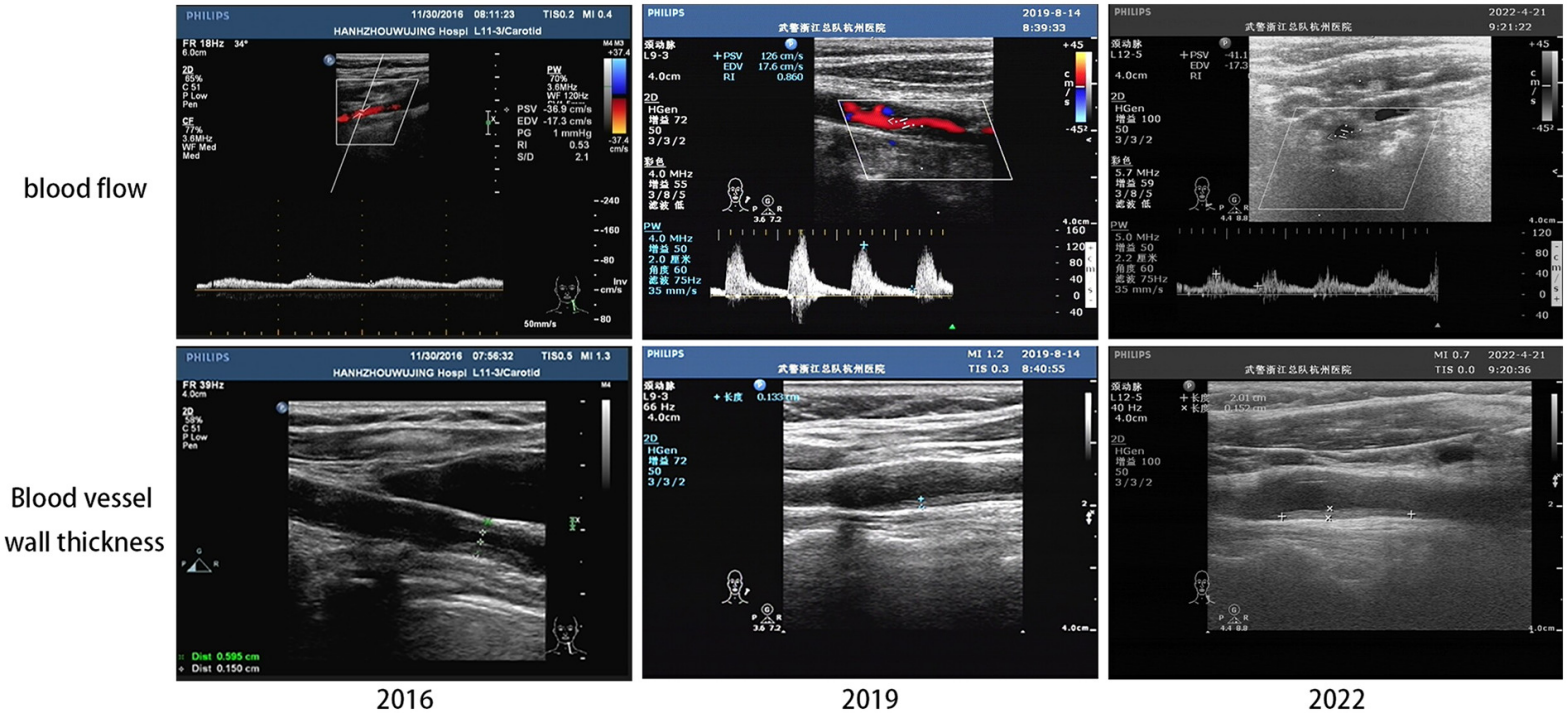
The follow-up results of the ultrasound of the left carotid artery. The specific area of measurement (upper, white box) and the specific point of measurement of blood vessel thickness (lower, red arrow) can be seen in the above ultrasound image.
